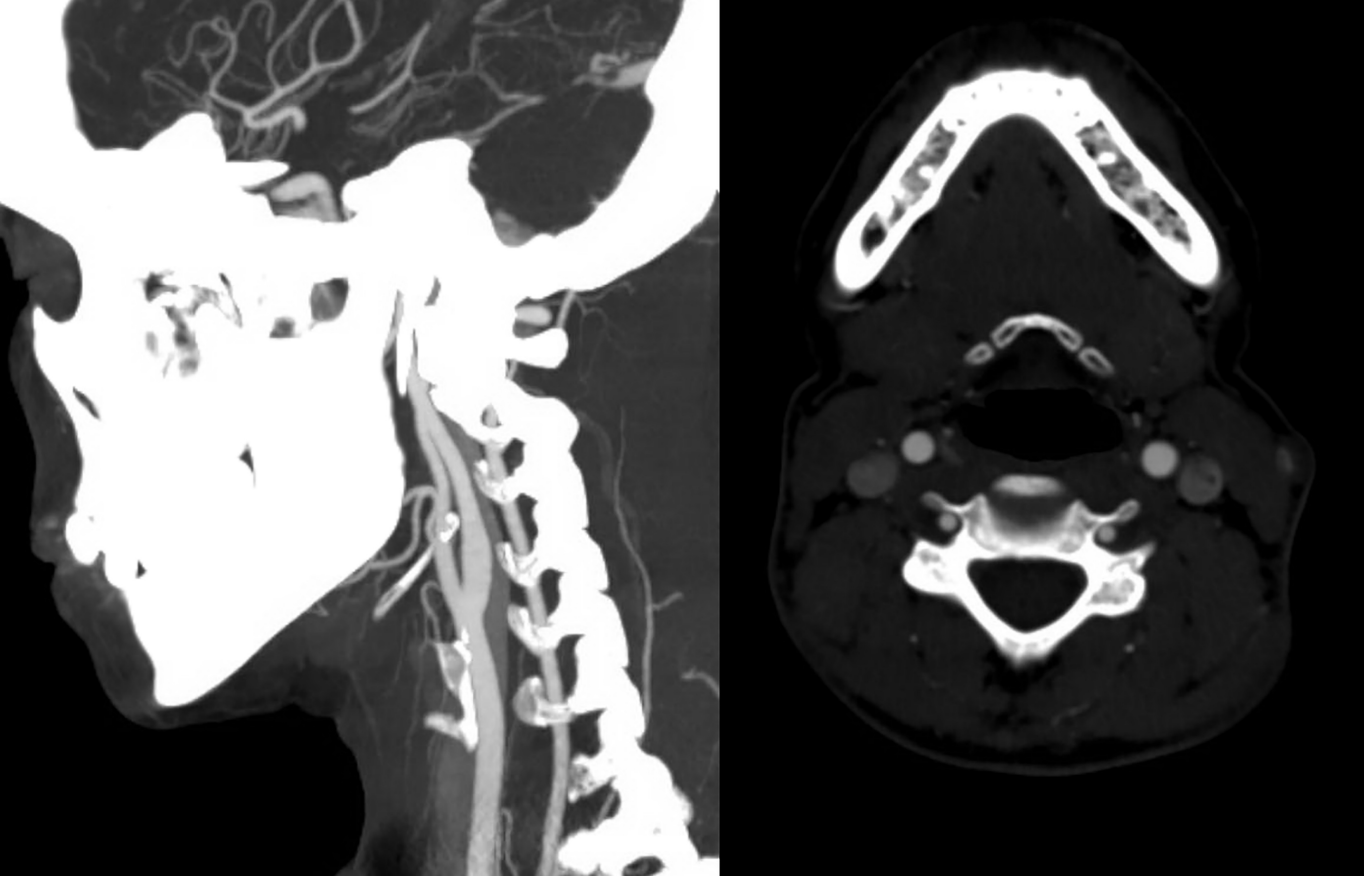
Sagittal and axial CT angiogram images demonstrate normal vertebral arteries without evidence of dissection
